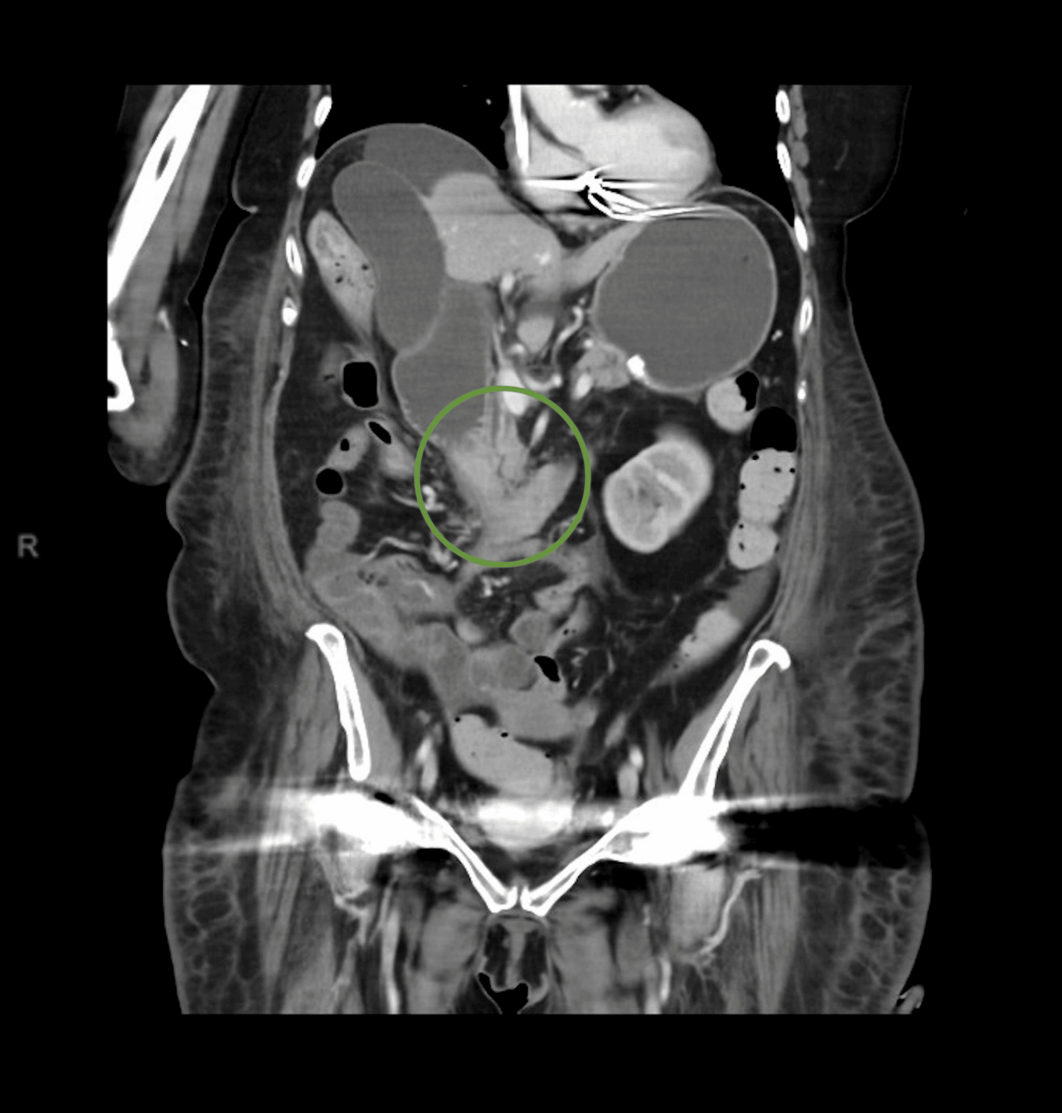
CT of the abdomen and pelvis (coronal view) showing the marked distension of the stomach and proximal D2 duodenum, with collapsed D2/D3, which was tethered secondary to retroperitoneal fibrosis CT: computed tomography
